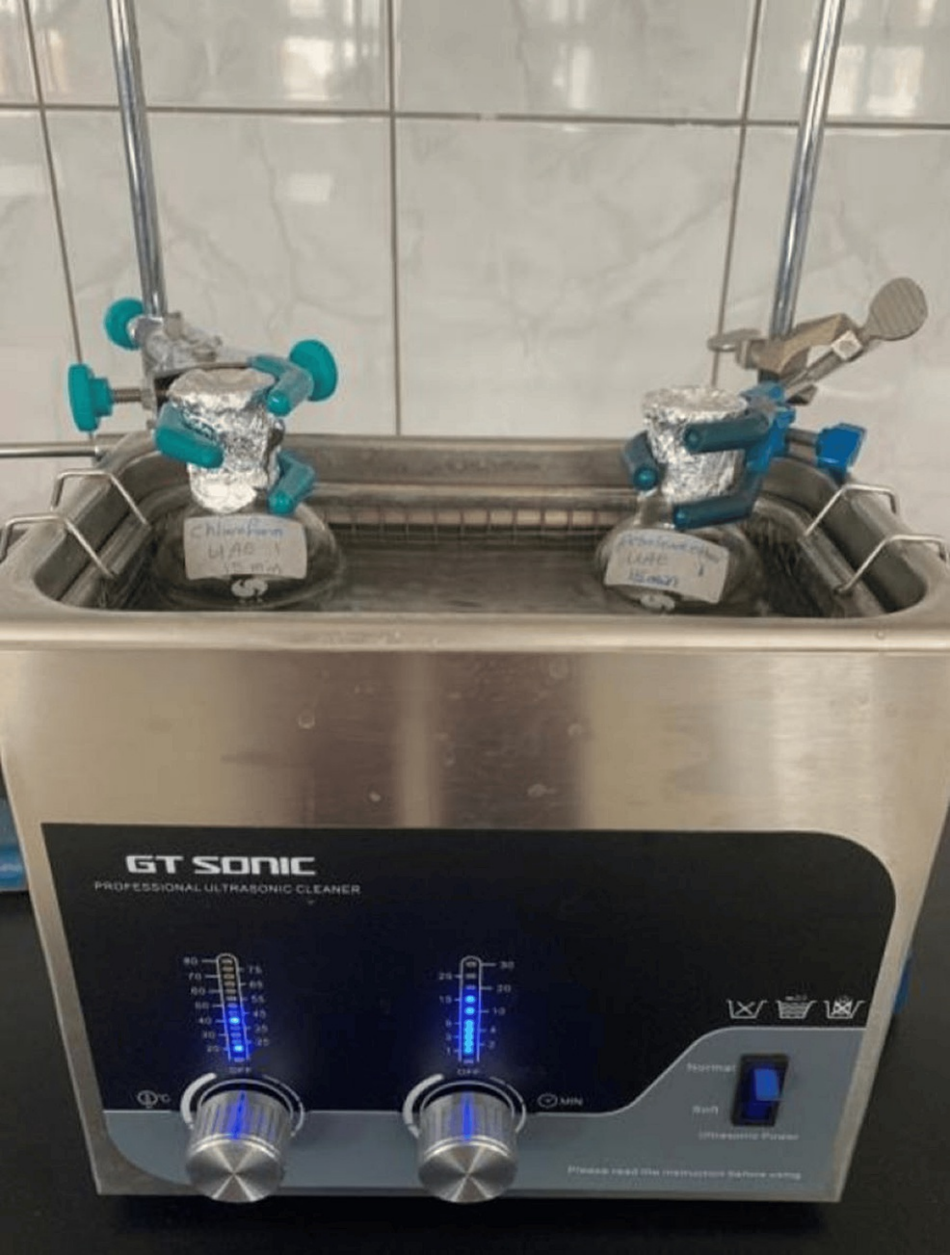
Ultrasound assisted extraction of Urtica dioica leaves and stems using (petroleum ether, chloroform, ethanol, and distilled water) solvents.
